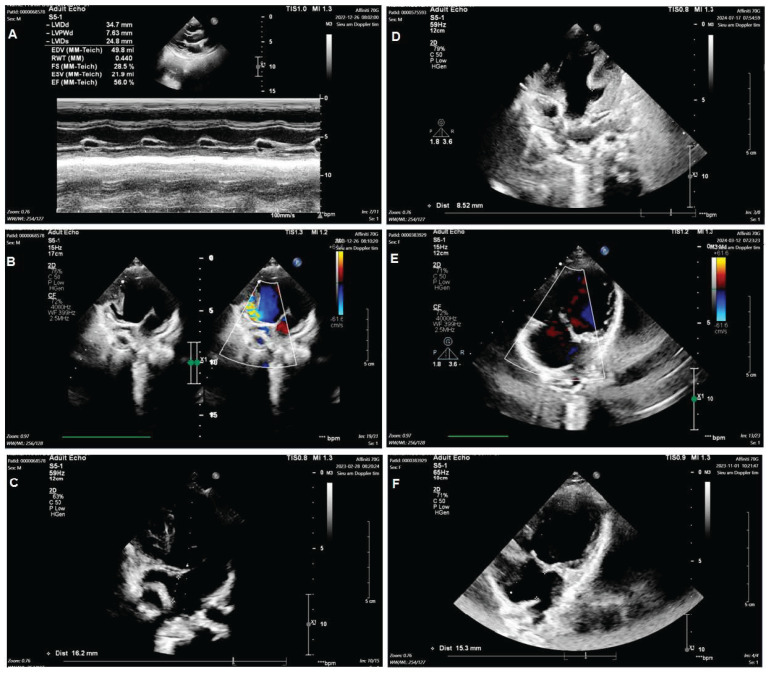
Echocardiogram in the patient with tricuspid valve atresia and in the patient with mitral valve atresia. Including the image of Tricuspid valve atresia (A); Ventricular septal defect (B); Atrial septal defect (C); Mitral valve atresia (D); Ventricular septal defect (E); Atrial septal defect (F) in patient.
